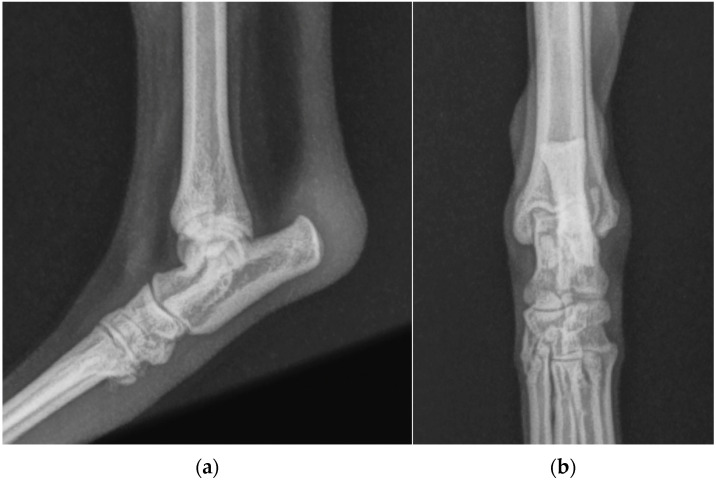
(a) Mediolateral and (b) dorsoplantar radiographic projections of the right tibial-tarsal joint’s highlighted radiopacity of pericalcaneal soft tissue without osteoarticular changes.
